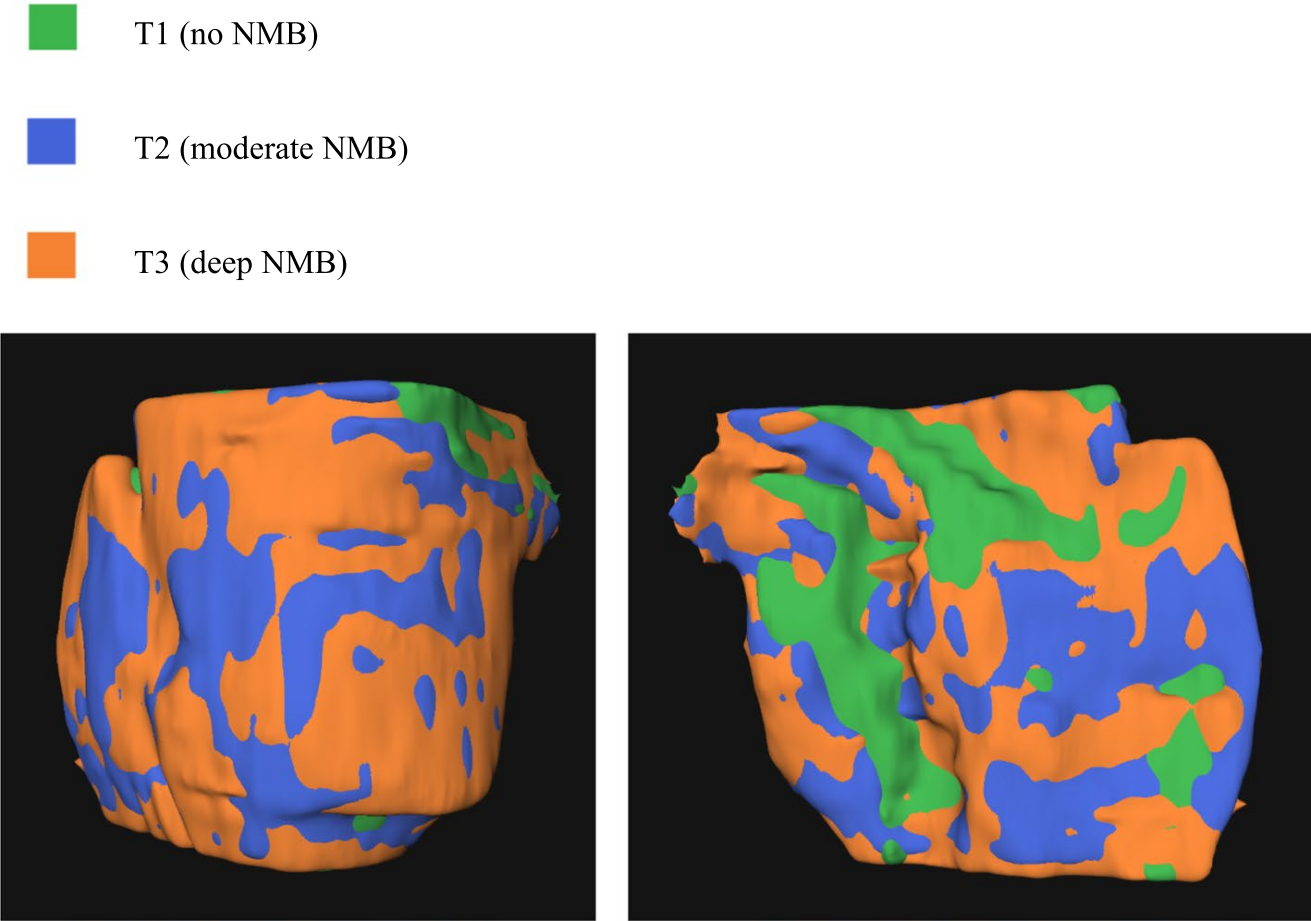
Overlapping 3D reconstructions of pneumoperitoneum shape in patient 10. In each slide of the MRI scans the insufflated area within the peritoneum was manually marked. When combined, a 3D shape could be reconstructed per scan. By overlapping the three 3D reconstructions of the pneumoperitoneum shape the direction of the volume changes could be visualised. Left image: ventral view. Right image: dorsal view
